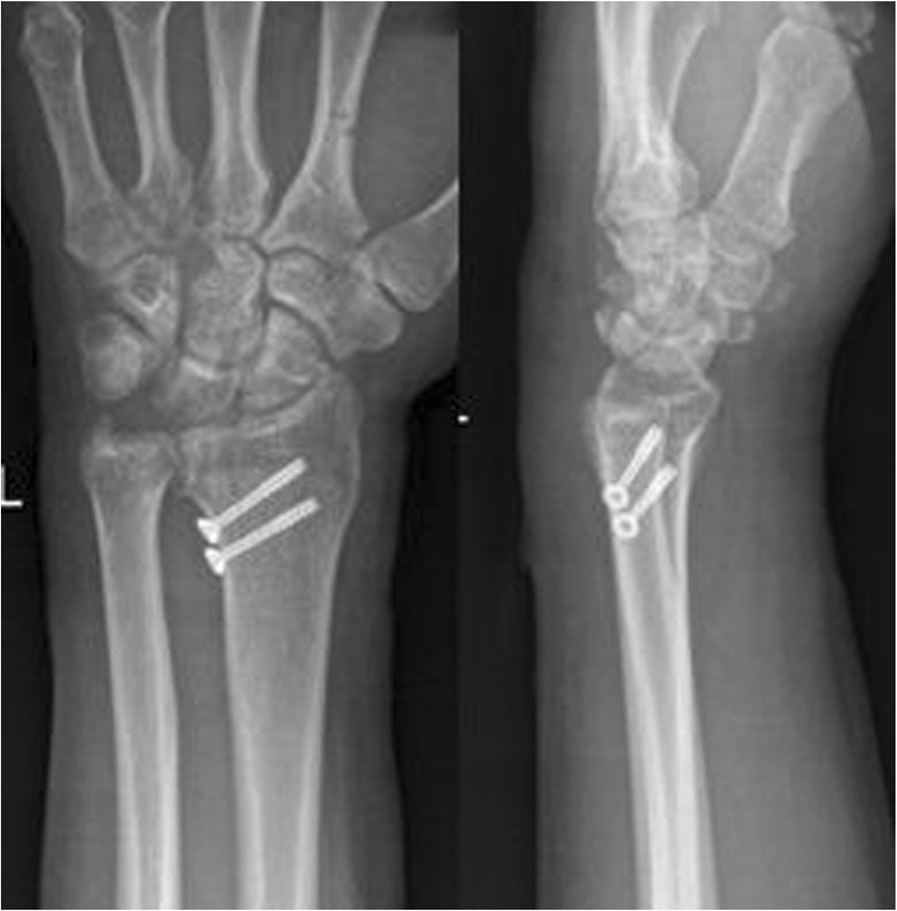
Post-operative X-ray film of ICF
